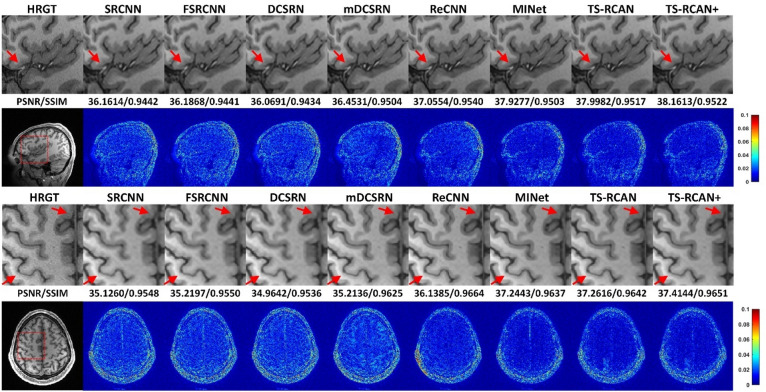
Qualitative comparison of 3D MRI SRR to the state-of-the methods with the scale factor of $$2\times 2\times 2$$. The SR images of SRCNN, FSRCNN and DCSRN exhibited noticeable blurriness in both the sagittal and axial views, leading to an almost complete loss of gray matter visibility in the hand knob region (indicated by the arrow). In contrast, ReCNN, MINet, and TS-RCAN demonstrated comparable performance with reduced errors and improved differentiation of small anatomical structures (arrow).
